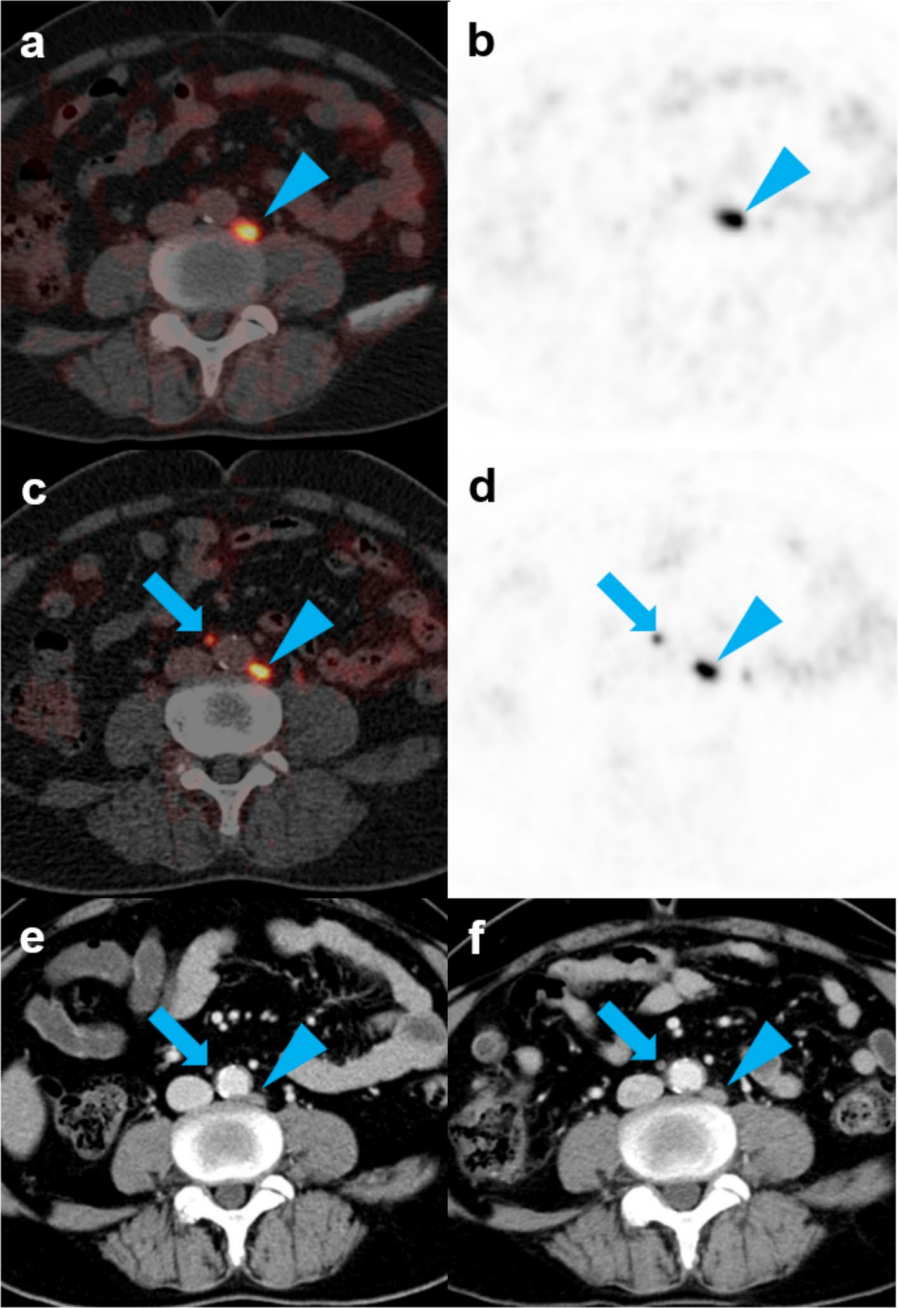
Detection of an additional metastatic lymph node by digital [^68^Ga]Ga-PSMA-11 PET/CT in a patient with prostate cancer who experienced BCR after prostatectomy. A 65-year-old man with prostate cancer was assessed by analog and digital [^68^Ga]Ga-PSMA-11 PET/CT after prostatectomy due to increased serum PSA level (5.02 ng/mL). (a,b) Analog PET/CT images showing a metastatic lymph node in the abdominal paraaortic area (arrowheads). (c,d) Digital PET/CT images showing a second small metastatic lymph node in the aortocaval area (arrows). (e) Subsequent contrast-enhanced CT, showing small lymph nodes in both the abdominal paraaortic and aortocaval areas. (f) Contrast-enhanced CT 6 months later showing an increase in size of both lymph nodes (arrows and arrowheads).
